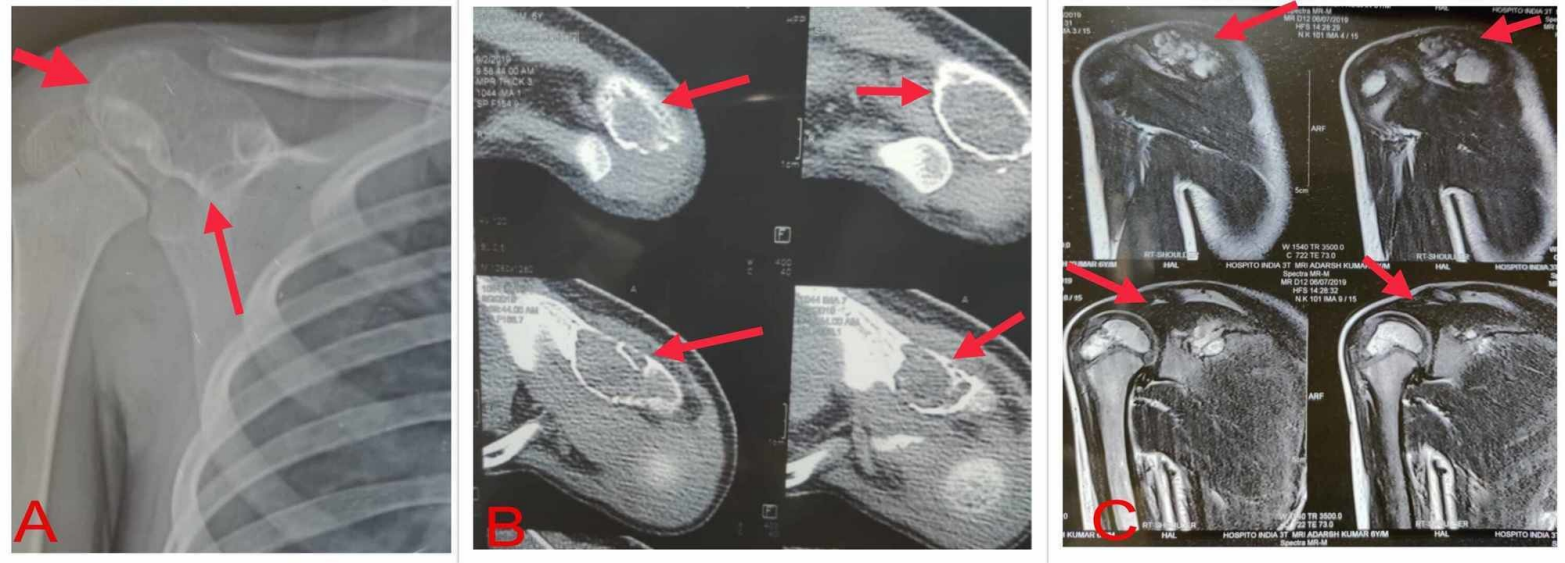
Preoperative radiographic assessment (A) X-ray showing an expansile lytic lesion in the acromion; (B) CT scan showing cortical breach and incomplete septations; (C) MRI showing the lesion to be hypointense on T1 and hyperintense on T2.
